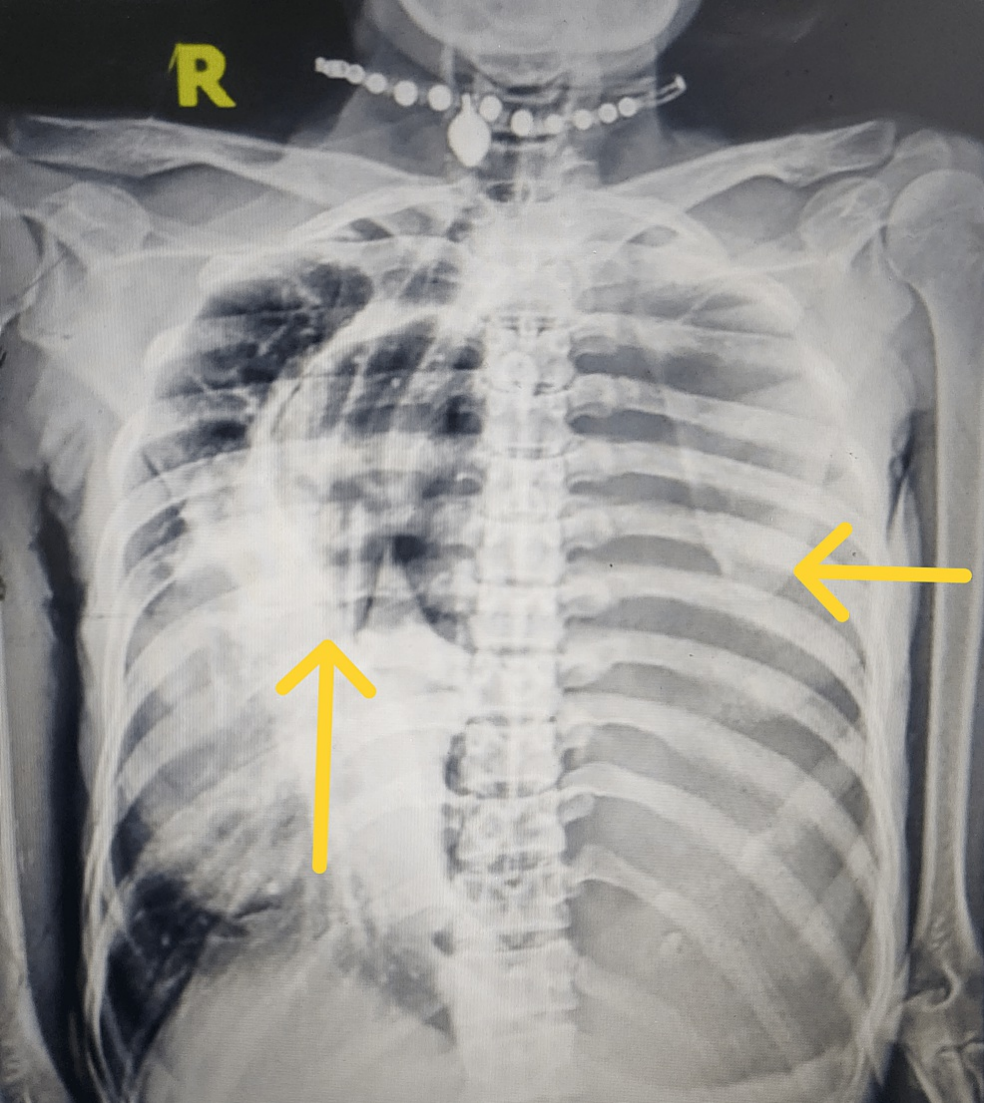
A supine anteroposterior chest X-ray on admission was suggestive of left-side massive hydropneumothorax with a shift of the mediastinum to the right.
